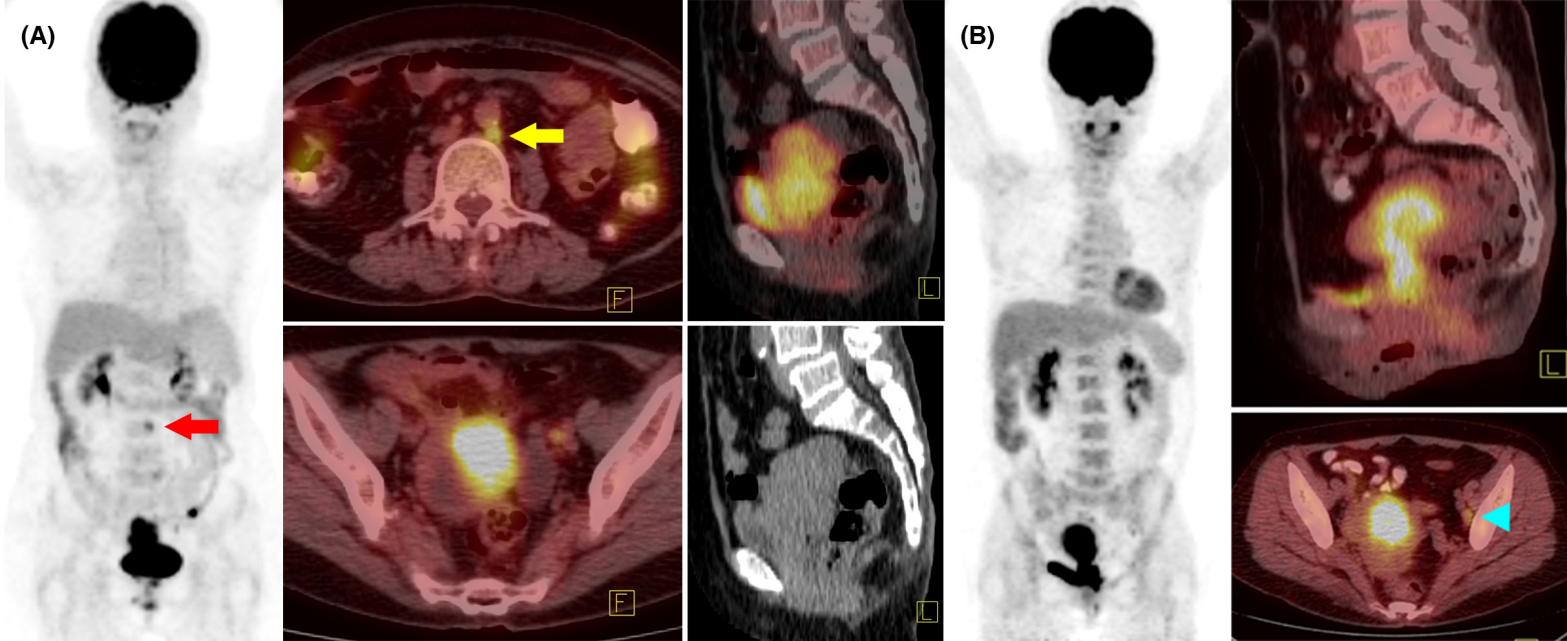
Representative cases of different survival rates in patients with different cumulative risk scores. (A) This is the case of a 79‐year‐old patient with FIGO 2009 stage IIIB cervical cancer. Pretreatment PET/CT revealed a bulky primary tumor (SUV_max_ = 14.8; MTV = 94 mL) with small positive pelvic and para‐aortic nodes (arrows). This patient had a cumulative risk score of 3 and received CCRT with cisplatin and bevacizumab. However, PET/CT imaging performed 3 months after the completion of CCRT revealed supraclavicular nodal metastases; nevertheless, cervical tumor and pelvic/para‐aortic lymph nodes indicated prominent regression. The patient died after another 3 months. (B) This is the case of a 57‐year‐old patient with FIGO stage IIIB cervical cancer. Pretreatment PET/CT revealed a cervical tumor with parametrial extension (SUV_max_ = 13.9; MTV = 85 mL) and a positive left external iliac lymph node (arrow head). This patient had a cumulative risk score of 0 and received standard CCRT with weekly cisplatin. The patient remained disease‐free for 3 years as of the latest follow‐up.
